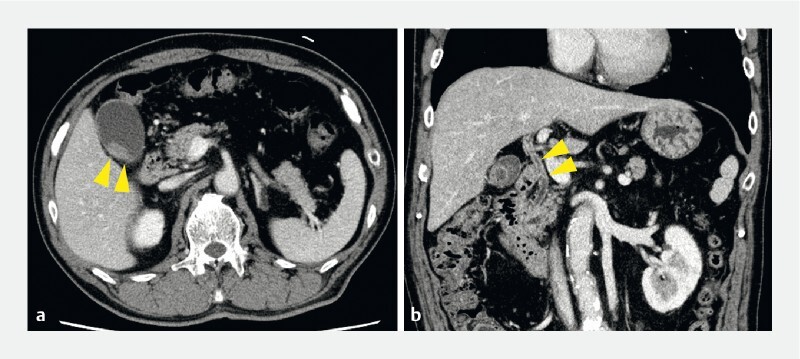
Contrast-enhanced computed tomography revealed thickening of the bile duct wall and localized wall thickening of the gallbladder fundus (arrows).
